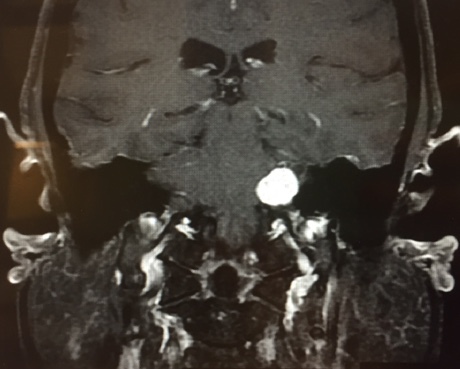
Figure 1 Acoustic Neuroma MRI brain/internal auditory canals of 19mm enhancing Ieft acoustic neuroma. Image obtained from North Oakland Ear, Nose & Throat Centers P.C.
